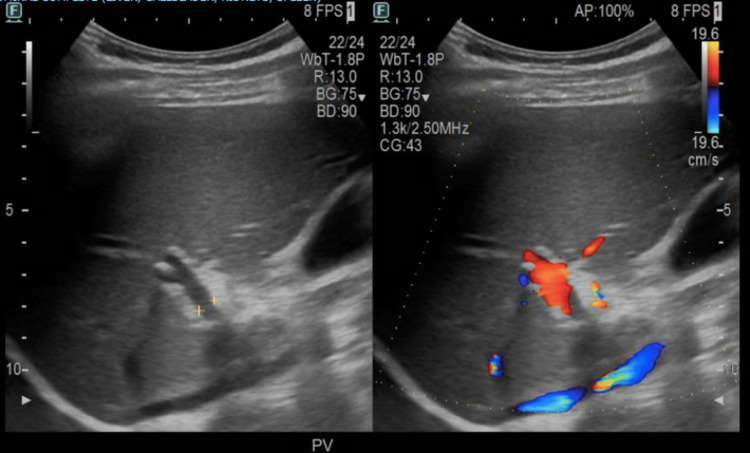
Ultrasound of the abdomen showing resolved venous thrombus in the portal vein
